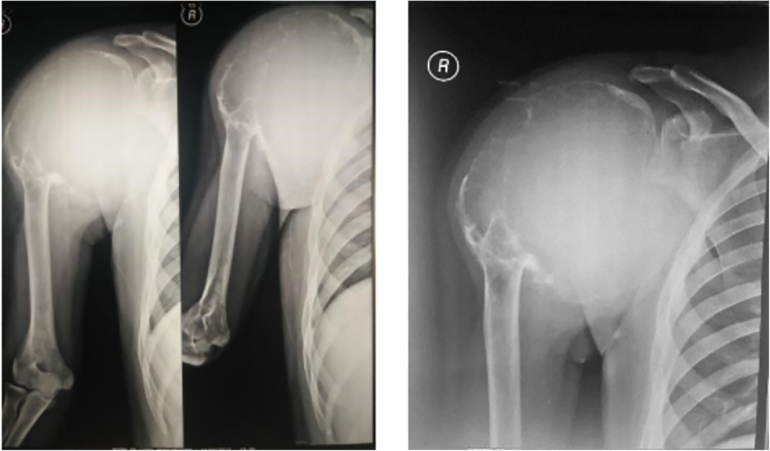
Anteroposterior and Lateral radiograph of shoulder depicts soft tissue tumor.
